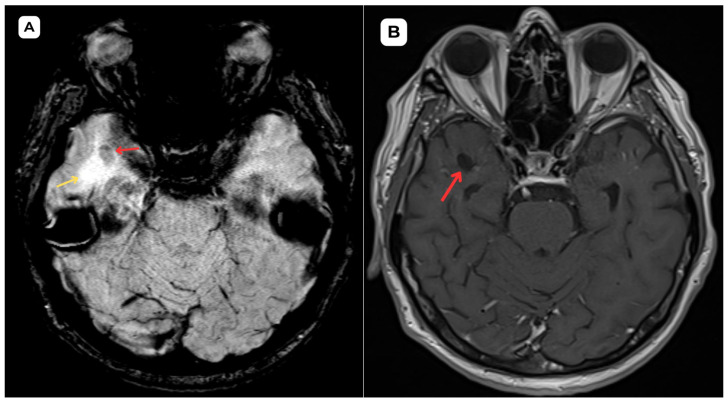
First patient: Brain MRI of a 63-year-old male. (A) SWI—susceptibility-weighted imaging, axial. No evidence of blood products or hemosiderin is seen, providing evidence of the non-hemorrhagic, benign nature of the cystic lesion. (B) The T1 axial post-contrast sequence shows no abnormal contrast enhancement in the cystic lesion, with no features suggestive of malignancy or disruption of the blood–brain barrier. Red arrow—opercular (type IV) perivascular space; yellow arrow—surrounding edema. In magnetic resonance imaging, the signal intensity of the opercular perivascular spaces themselves is identical to that of cerebrospinal fluid in all sequences—hypointense on T1W1 and FLAIR, hyperintense on T2W1, and the ADC value corresponds to cerebrospinal fluid [6,7]. In the images, these PVSs are located very close to the branches of the middle cerebral artery, which come into contact with the cerebral cortex, with MRI showing regional cortical thinning in the brain [6,7]. The presence of cerebrospinal fluid intensity tracts in the images is also useful as a radiological criterion for the diagnosis of perivascular spaces [1]. All of the above-mentioned features help distinguish opercular perivascular spaces from neuroglial tumors with characteristic perifocal edema.
